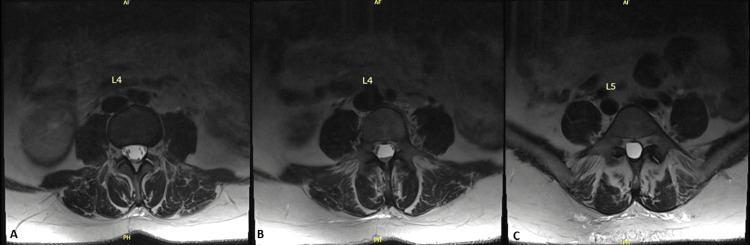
Initial MRI transverse view. From left to right, these images show the initial T2 MRI findings, which reveal peripheral displacement of the nerve roots. Image A is the superior portion of the 4th lumbar vertebra. Image B is the inferior portion of the 4th lumbar vertebra. Image C is the 5th lumbar vertebra. The transition of the images demonstrates an "empty thecal sac" appearance, which can be seen in patients with inflammatory arthropathy as well as arachnoiditis.
